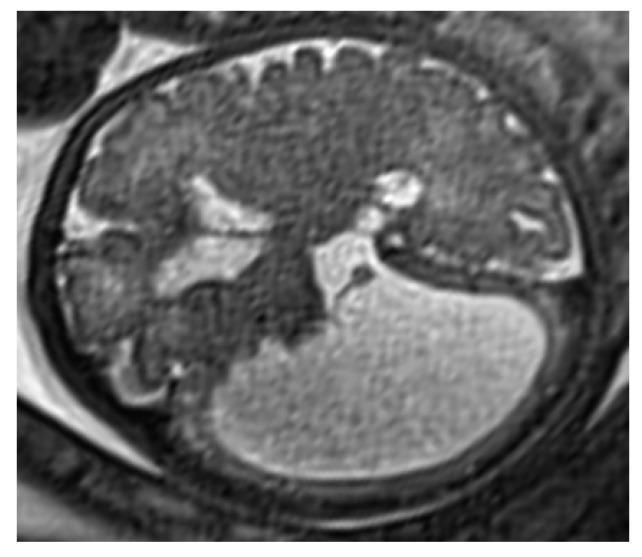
MRI picture of ventriculomegaly.
